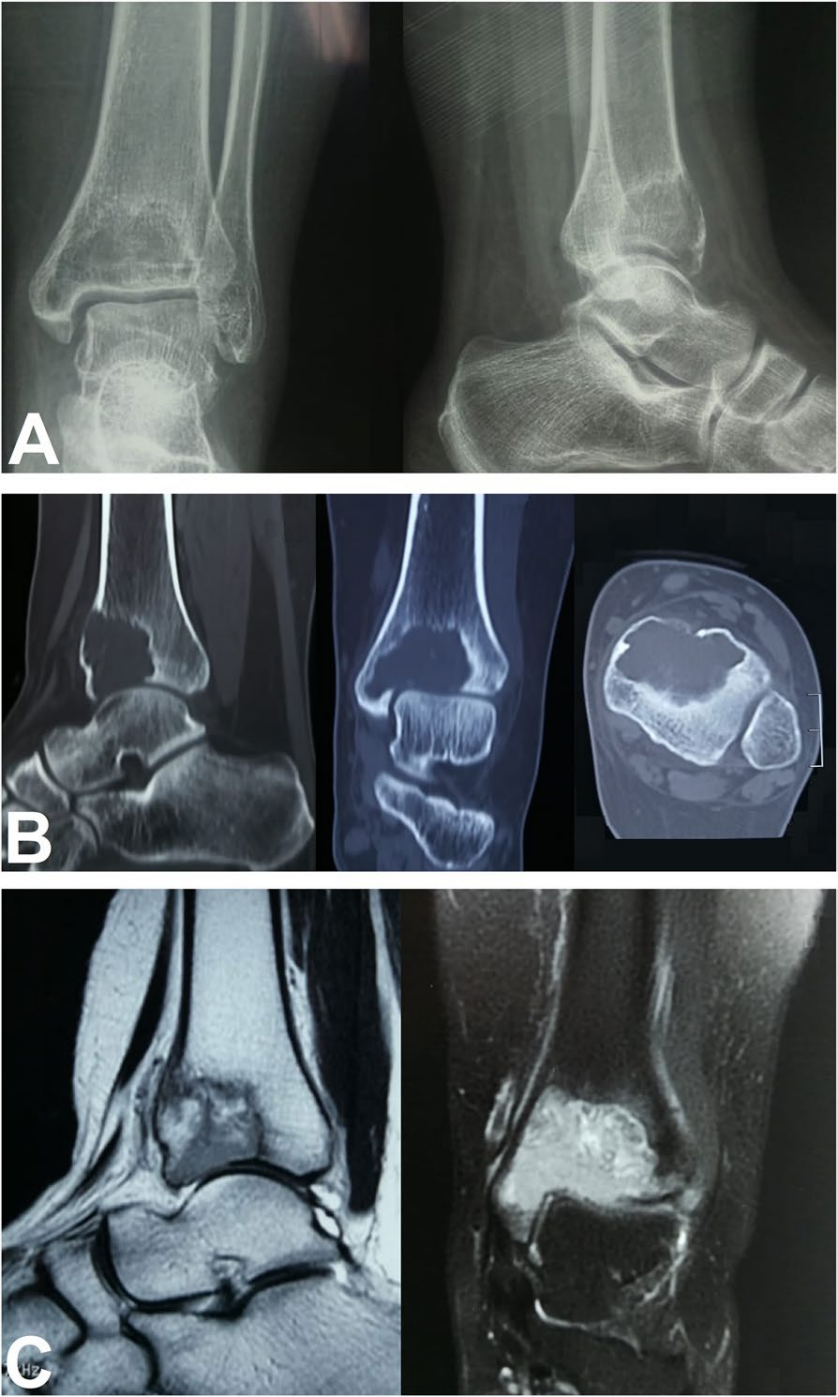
A 22-year-old female presented with limping, pain aggravated on walking, and restricted movement of the left ankle for 7 months. The patient had no history of trauma and no constitutional symptoms. Examination revealed tenderness over the anterior aspect of the distal tibia, with painful and restricted range of motion of the left ankle. A Preoperative anteroposterior and lateral X-rays showing a well-defined expansile osteolytic lesion in the epi-metaphyseal region of the left distal tibia suggestive of GCTB. The tumor was classified as Campanacci grade III. B Sagittal, coronal and axial CT scans showing an osteolytic lesion occupying the anterior two-thirds of the epi-metaphyseal region of the left distal tibia with erosion of the articular surface. C Sagittal and coronal MRI images showing an eccentric expansile osteolytic lesion measuring 4 (craniocaudal) × 3.5 (transverse) × 2.5 (anteroposterior) cm breaching the articular surface with only the posterior one-third of the tibial plafond preserved with no soft tissue or intra-articular extension
